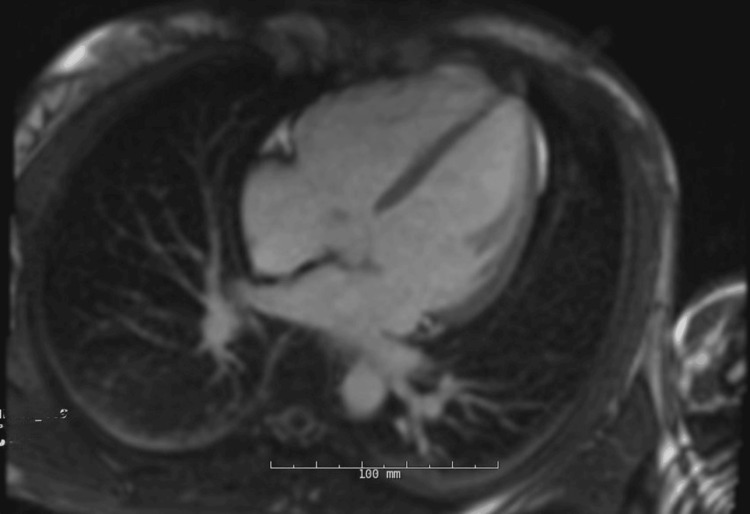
Cardiac magnetic resonance imaging. MRI demonstrating normal right and left ventricular size without evidence of fibrosis or scarring.
